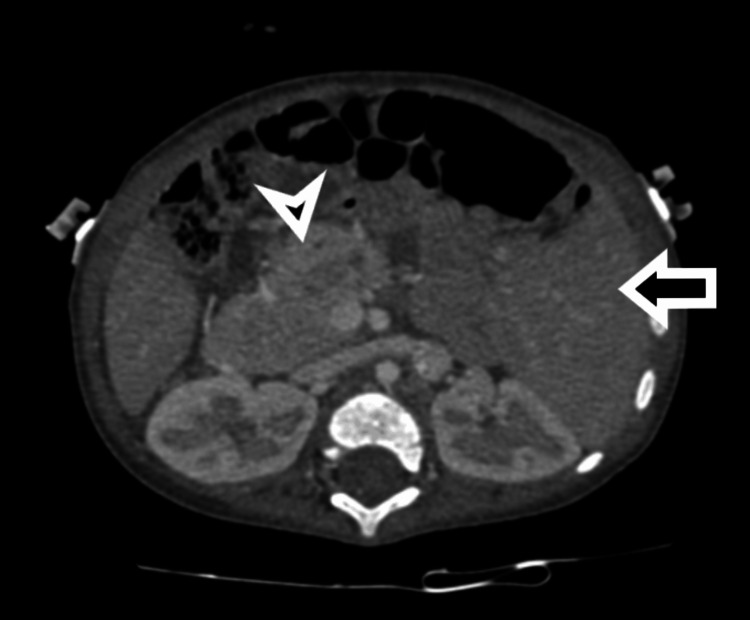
Contrast-enhanced computed tomography imaging of the abdomen revealing a central liver (arrow) with an annular pancreas (arrowhead).
